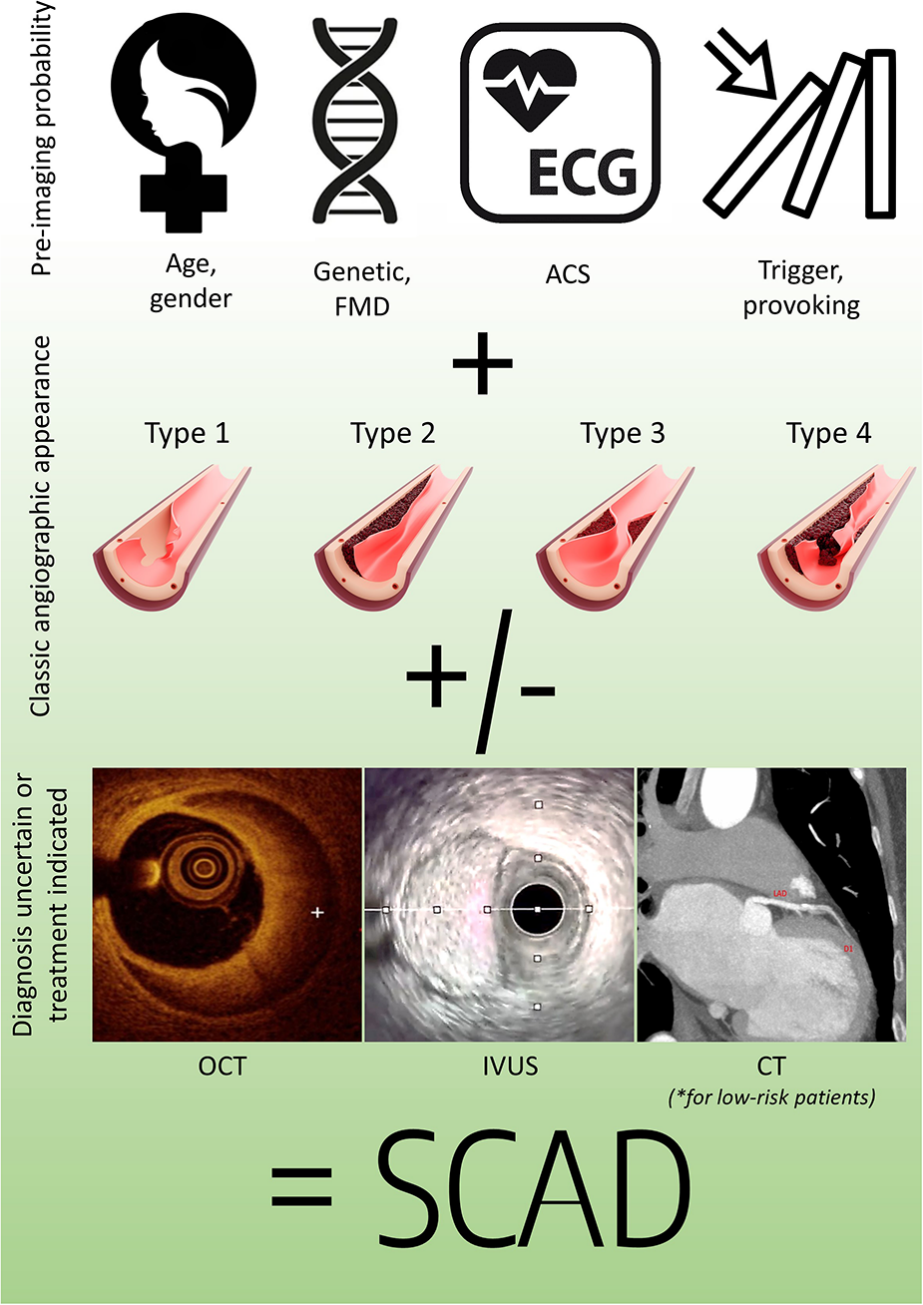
Holistic SCAD diagnostic pathway. ACS, acute coronary syndrome; CT, computed tomography; FMD, fibromuscular dysplasia; IVUS, intravascular ultrasound; OCT, optical coherence tomography.
